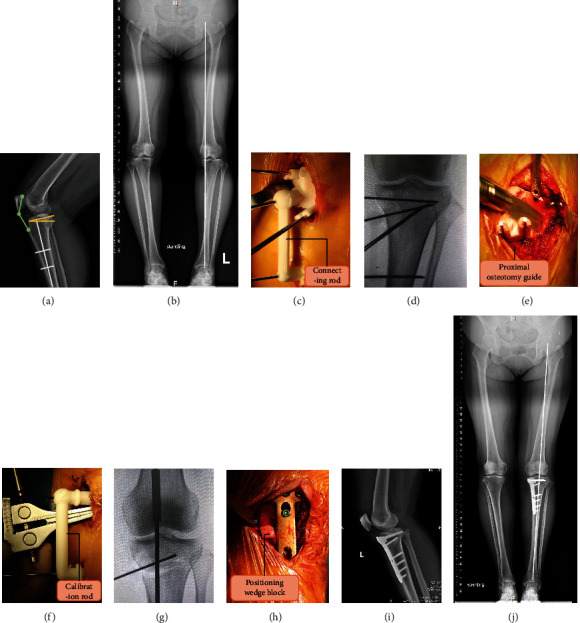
Surgical technique of PSI. (a, b) Preoperative imaging. The Insall–Salvati index is indicated by the green lines: ratio of the length from the inferior pole of the patella to the superior border of the tibial tuberosity and the longest diagonal length of the patella. The PTSA is indicated by the yellow lines. (c) Installation and fixation of the proximal osteotomy guide. (d) X-ray fluoroscopy to confirm the position of the guide. (e) Biplanar osteotomy according to the orientation of the osteotomy guide and Kirschner wires. (f) Calibration of the open wedge with the aid of calibratable rod. (g) Intraoperative radiography to assist in confirming the alignment of lower limb. (h) Installation of the plate fixator under the guidance of the positioning wedge block. (i, j) Postoperative imaging. (PSI: patient-specific instrumentation. PTSA: posterior tibial slope angle.).
